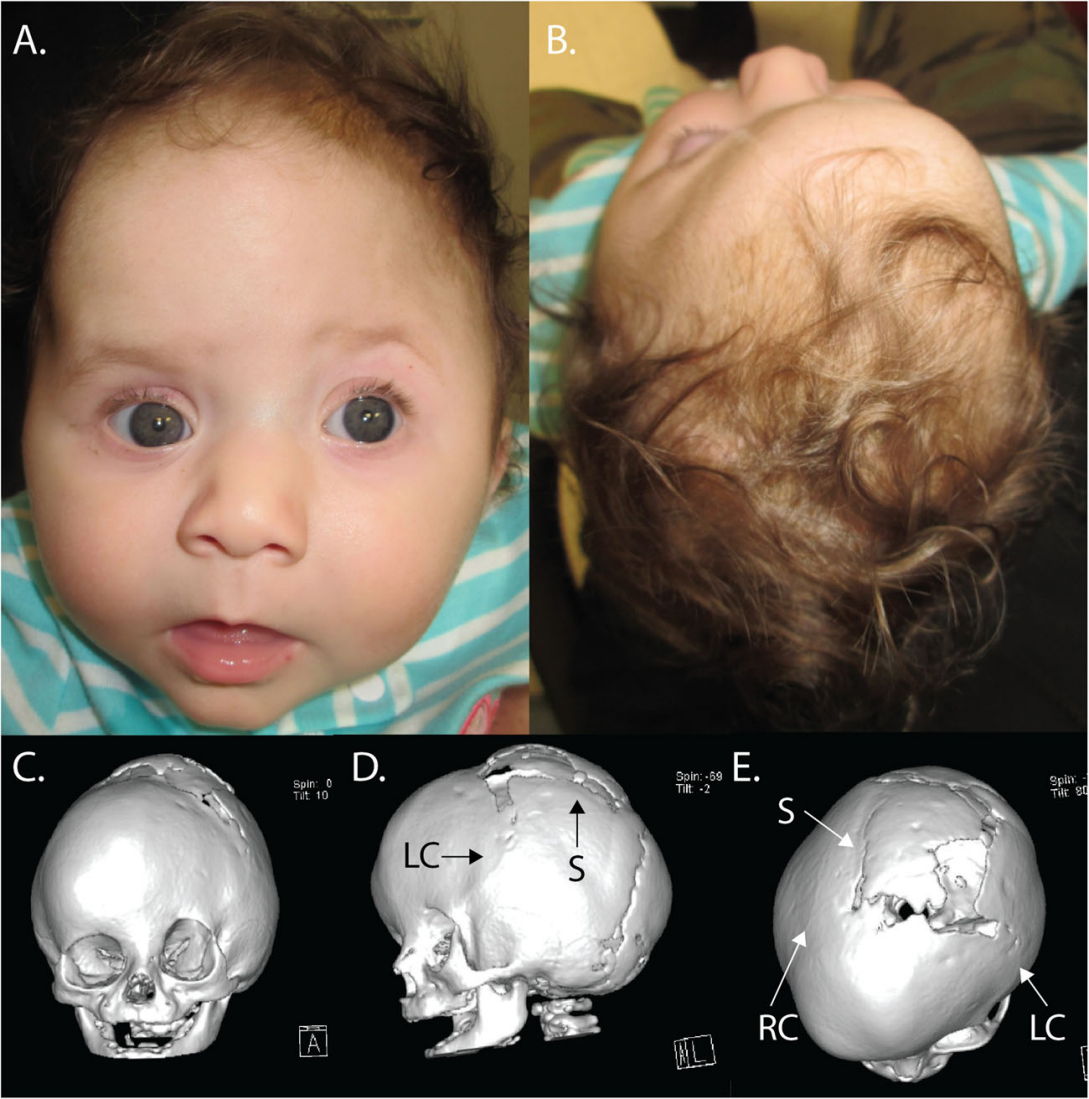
Patient photographs and 3D CT imaging from a second operation at 6 months of age. a Frontal view demonstrating left coronal synostosis with anterior plagiocephaly (suture fusion causing ipsilateral flattening and contralateral bossing of the forehead). Note the “twisted” appearance of the face as a result of unicoronal synostosis. b Anterior plagiocephaly as seen from above. Note the bossing of the right forehead and retrusion of the left forehead. Coronal (c), lateral (d), and axial (e) views of the 3D CT reconstruction performed three months after the initial strip craniectomy (age 5.5 months), demonstrating fusion of both the right coronal (RC) and left coronal (LC) sutures and sequelae of the previous strip craniectomy (S)
